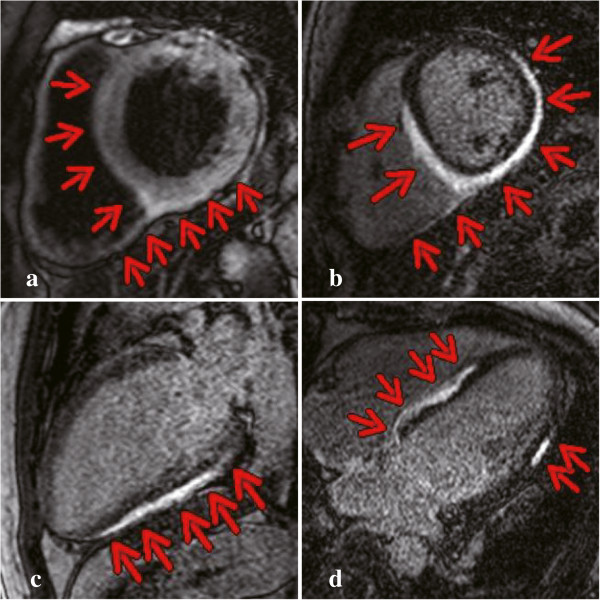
Cardiovascular magnetic resonance imaging. (a) T2-weighted imaging showing enhancement in the septum and posterior wall. (b–d) Late gadolinium enhancement showing subepicardial enhancement in the septal, anterolateral, and posterior walls.
